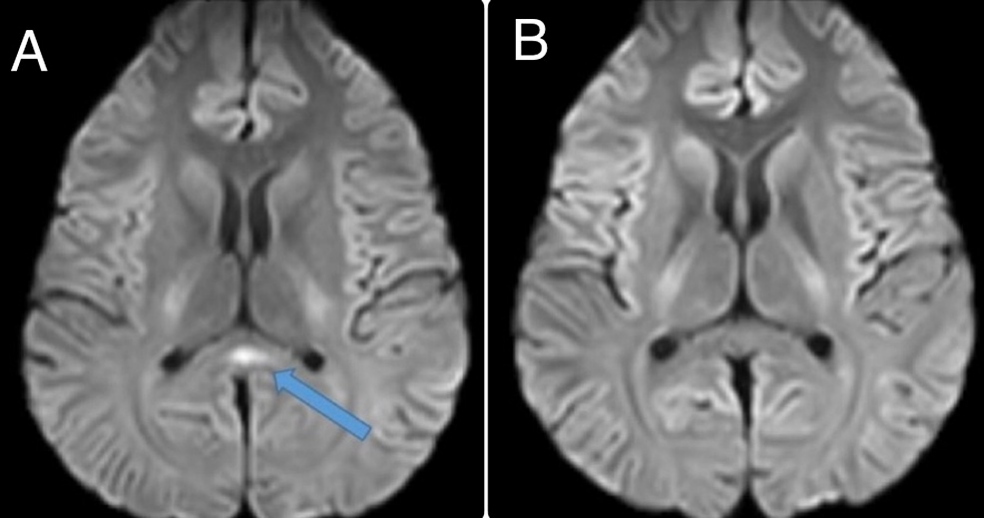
MRI brain showing the boomerang sign and its resolution after treatment. (A) First MRI showing a small splenial diffusion-weighted MRI hyperintensity (blue arrow). (B) Repeat MRI done after one week shows resolution of splenial hyperintensity.
